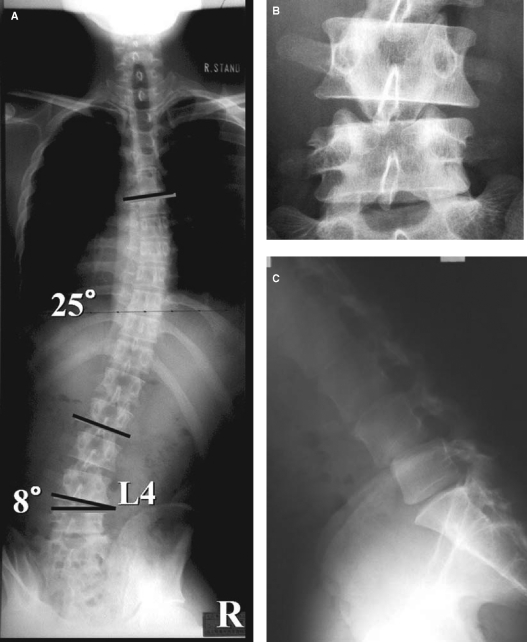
Preoperative plain X-rays. A: Posteroanterior view of the full spine in upright position revealed wedging of the L4/5 disc and the compensatory scoliosis in the cephalad portion of the spine. B: Posteroanterior view of the lumbar spine revealed bilateral spondylolysis of the inferior articular processes of the fourth lumbar vertebra. C: A lateral view of the spine revealed anterolisthesis of the L4 vertebra and a significant pathological opening of the posterior disc space at L4/5 in flexion position.
